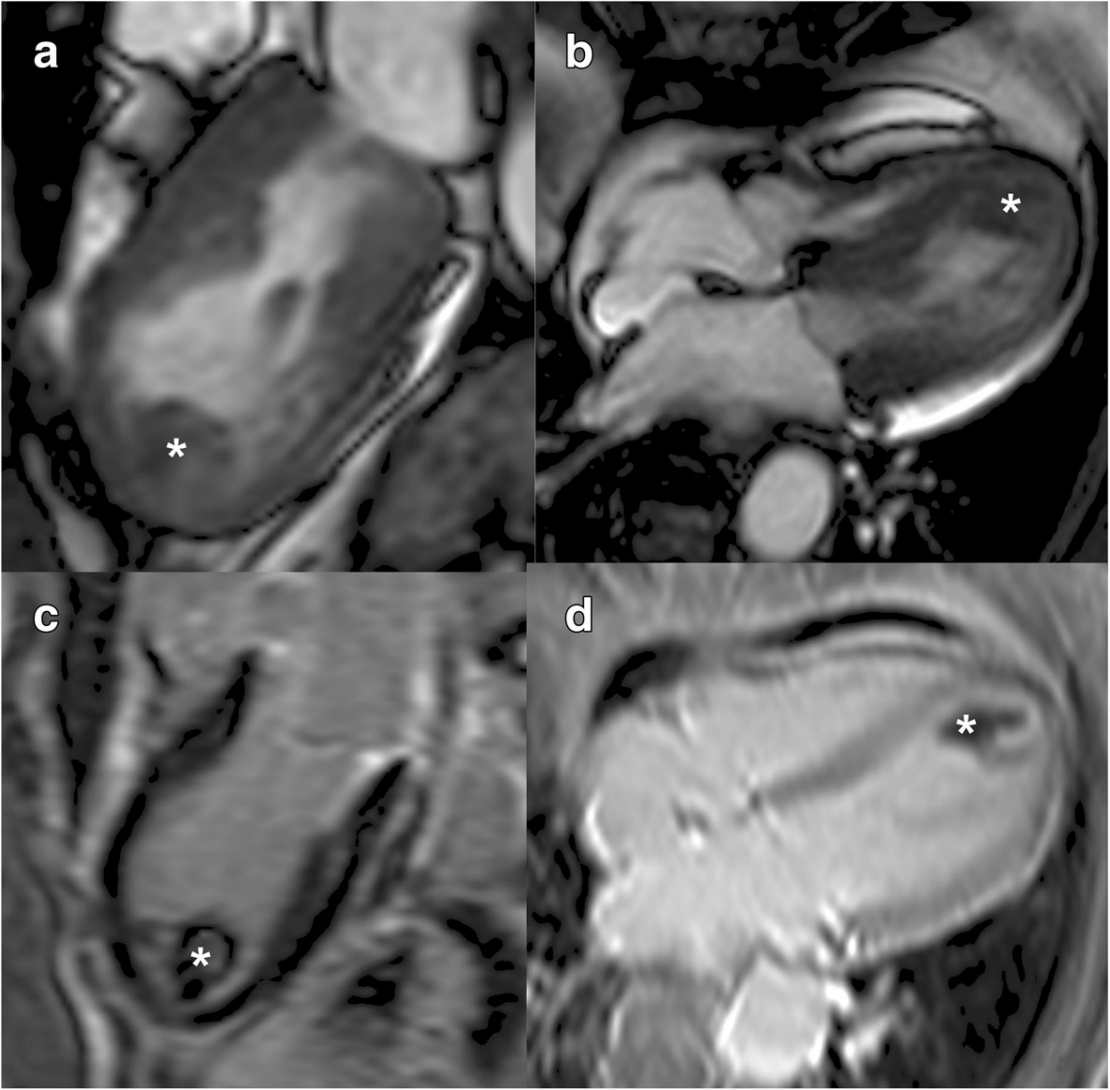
Cardiovascular magnetic resonance imaging of a patient with apical ballooning due to Takotsubo Syndrome. Apical left ventricular thrombus formation (asterisks) in the end-systolic two-chamber view (a) and four-chamber view (b). Inversion recovery sequence shows a lack of Late Gadolinium Enhancement in the myocardial tissue (c) and confirms thrombus in the sequence with long inversion time (d)
